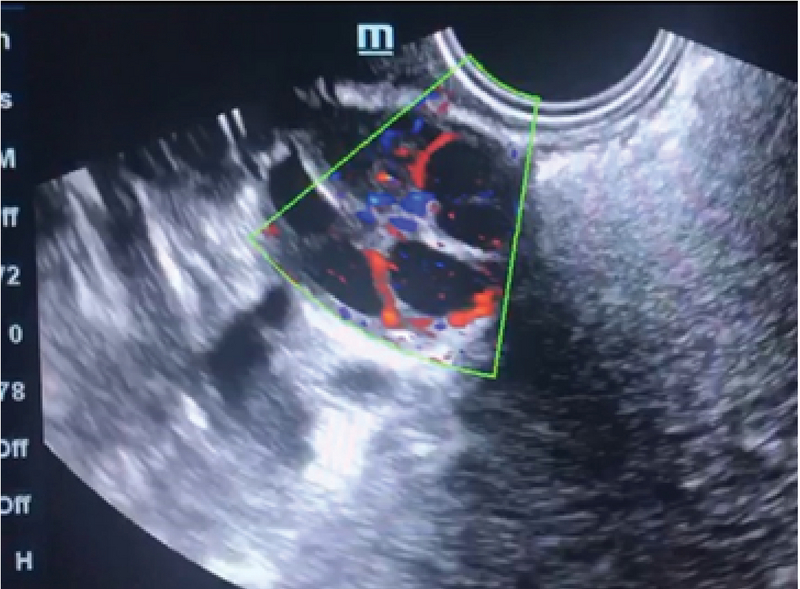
Perifollicular vascularity in 2D ultrasound with Color Doppler.
